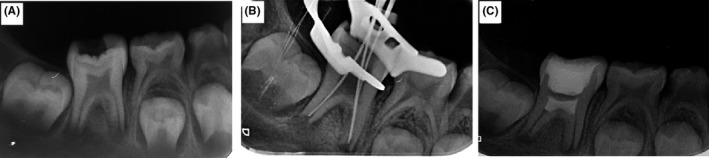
(A) Preoperative radiograph showing the right mandibular molar with periapical radiolucency, deep carious lesions, and PDL widening. (B) Working length determination and (C) postoperative final radiograph after induction of bleeding and MTA placement
